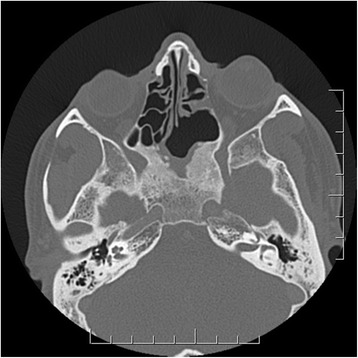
Axial computed tomography scanning of the brain demonstrating an asymmetric thickening in the *left* posterior cavernous sinus extending to the *right* anterior cavernous sinus and *right* orbital apex
